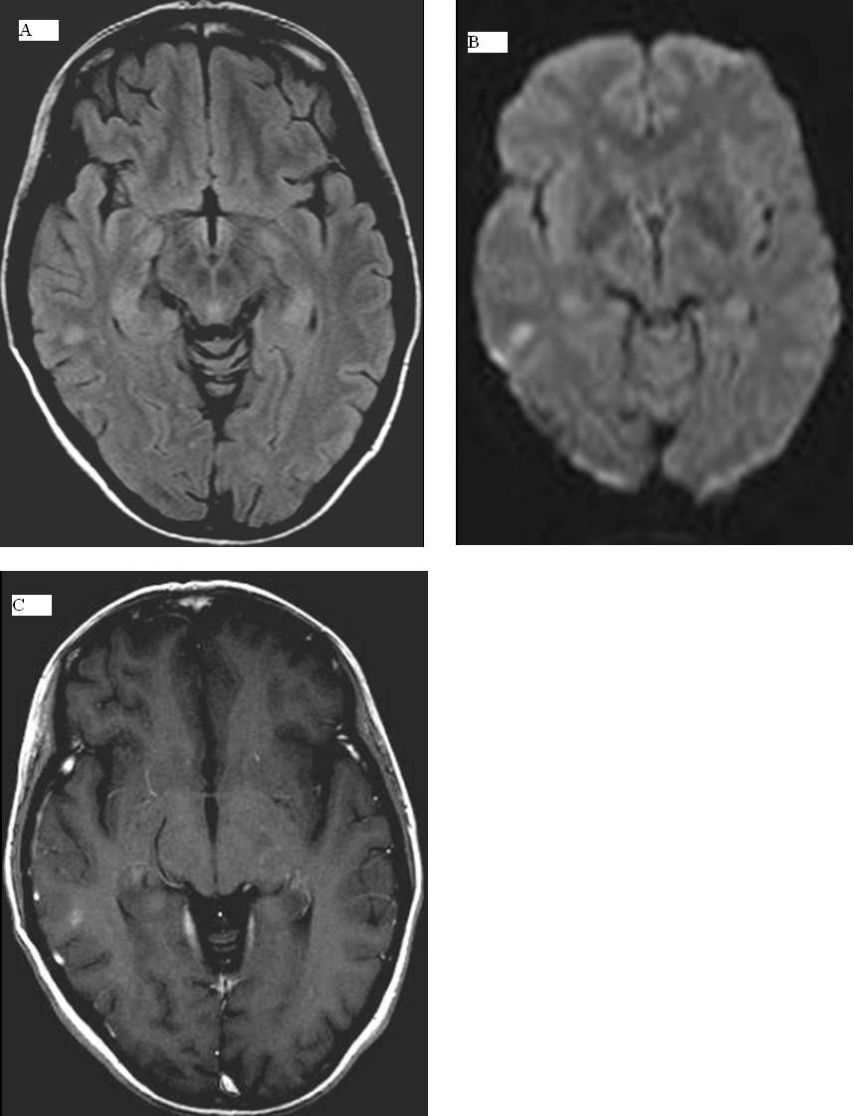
(A) Right temporooccipital lesion can be easily detected as a hyperintense spot in T1-weighted sequences . (B) The lesion shows restricted diffusion, an absence of perilesional edema. (C) No enhancement is observed after gadodiamide injection. These findings are suggestive of areas of microischemic phenomena.
